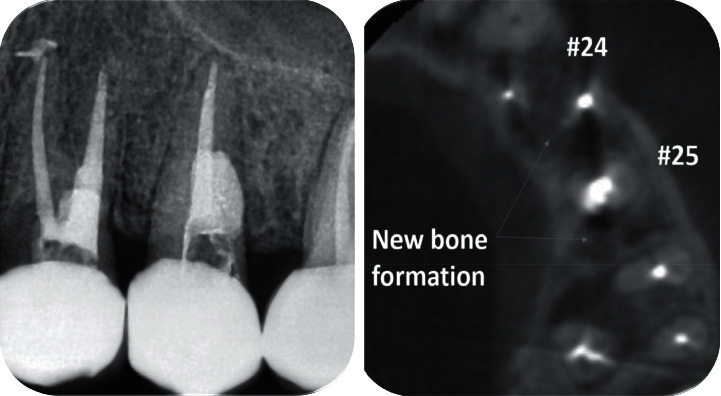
Periapical radiography and CBCT image after a 3-year follow-up.
